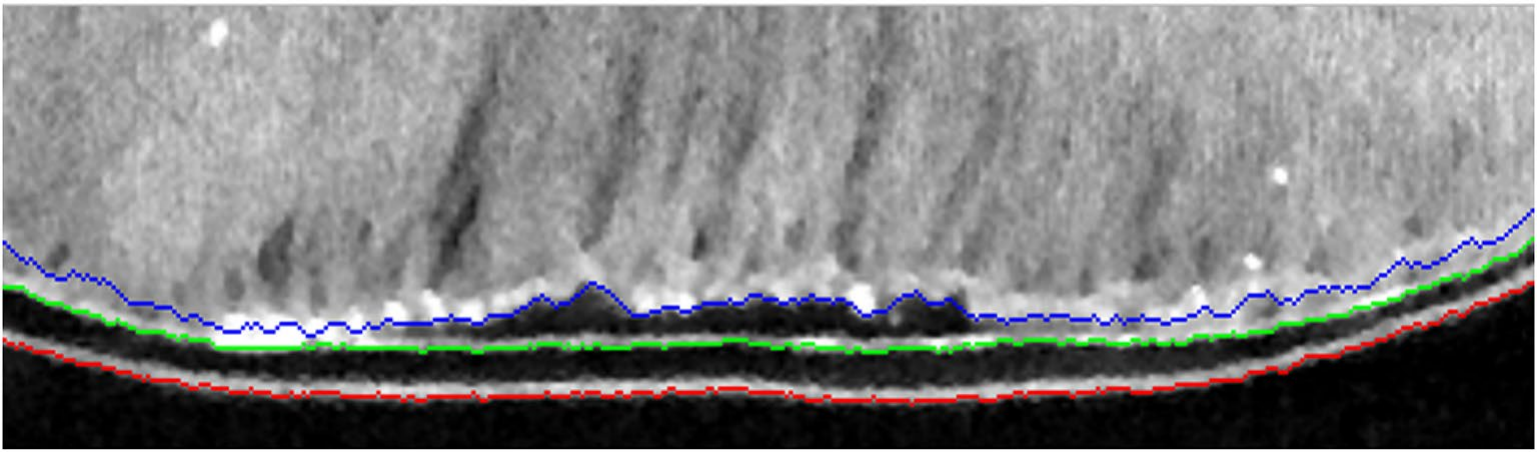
In this detailed view, one slice of the volumetric 3D CT image acquired of one volume is shown, in which the spine’s three layer surfaces have been segmented by LOGISMOS. The spine is formed of the gatherings of folded paper sheets of the book’s textblock (blue); parchment spine patches placed on the spine (green); and tawed pigskin cover (red)
